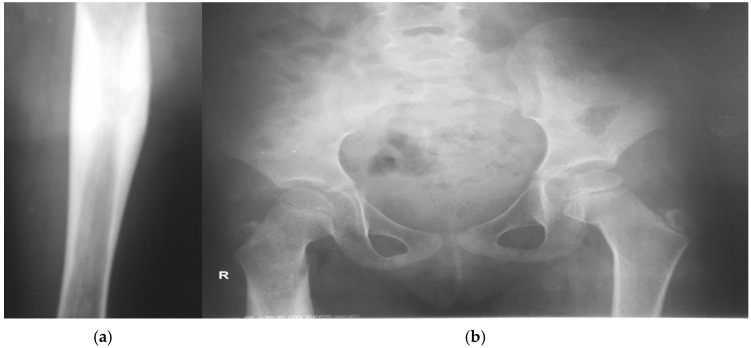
Radiography findings of the right femur: (a) cortical thickening and periosteal reaction along the diaphysis; (b) osteoporotic changes involving the femoral head, greater trochanter, and acetabulum.
